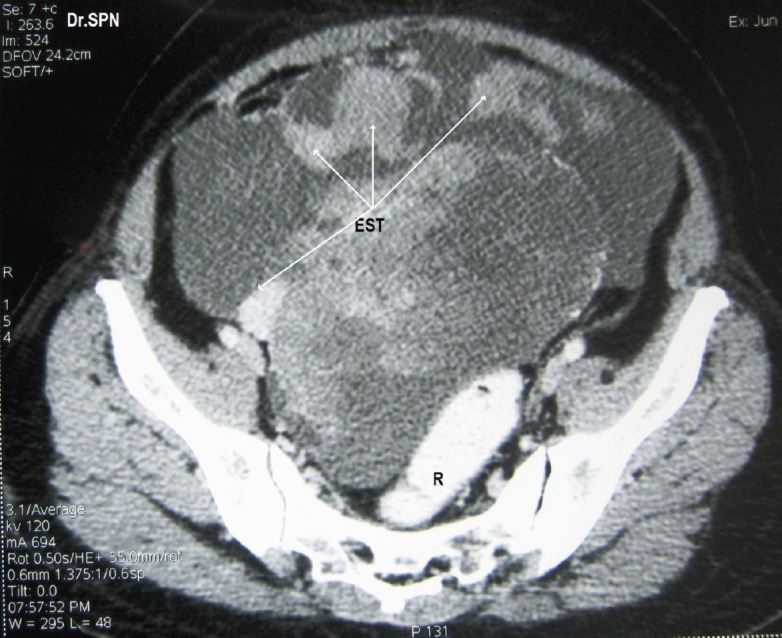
CT scan with oral and rectal contrast (R) showing a pelvic mass with multiple heterogeneously enhancing soft tissue masses with central necrotic areas within pelvis not separately seen from the ovaries (EST).
